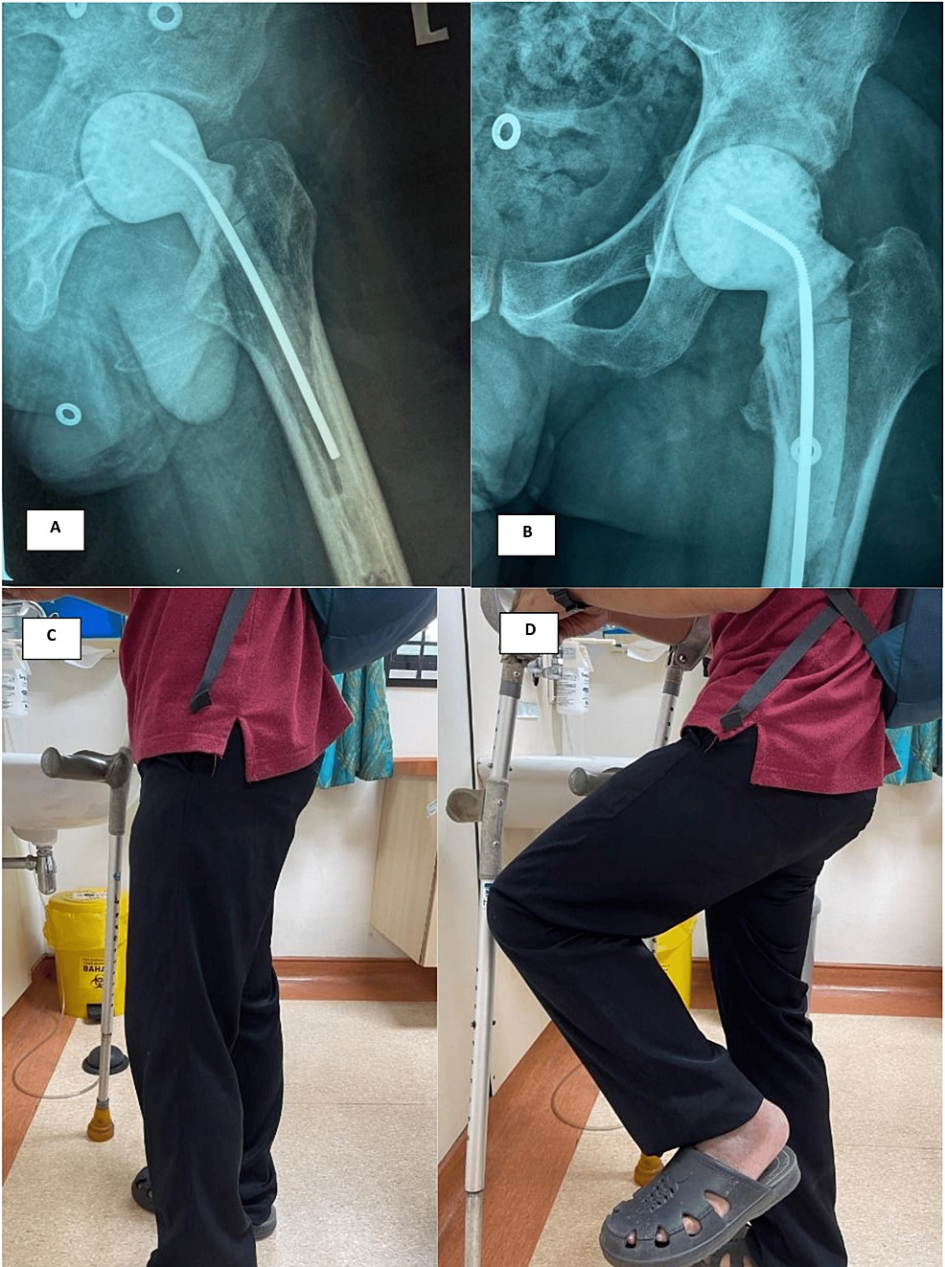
(A) Post-operative plain radiograph in lateral view. (B) Post-operative plain radiograph in AP view. (C) The patient was able to weight-bear with aid. (D) Hip flexion is up to 70 degrees. AP: anteroposterior
